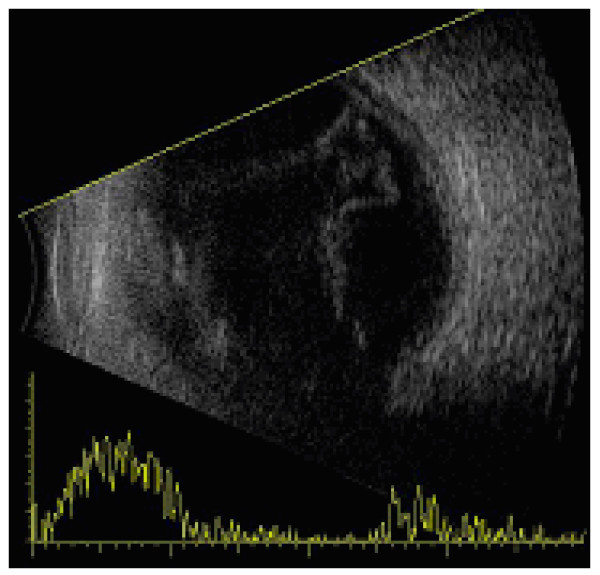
B-scan ultrasonography showing evidence of vitreous abscess with an intact retina in his right eye
